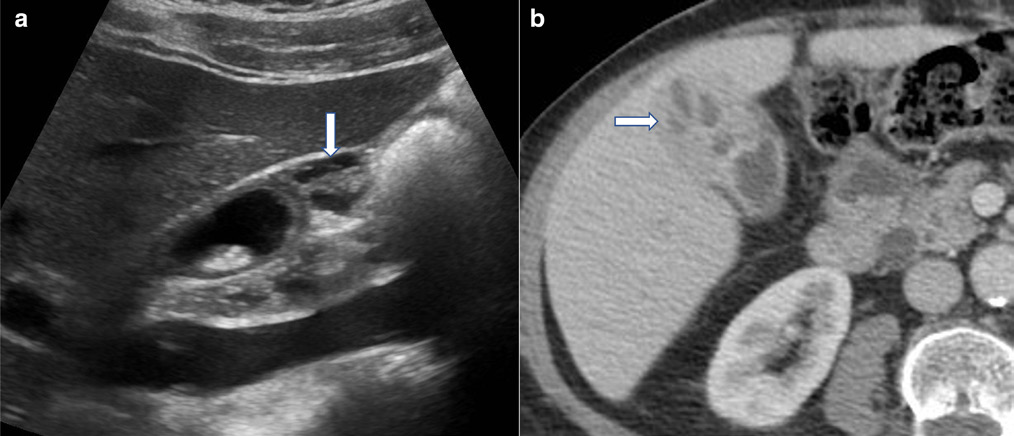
(a) Greyscale ultrasound showing anechoic cystic spaces in a thickened gallbladder fundus consistent with adenomyomatosis. (b) Axial contrast-enhanced CT also demonstrating cystic spaces within a thickened gallbladder fundus. Note the gallbladder mucosa enhances normally and appears intact. The cystic spaces are usually a sign of a benign process and are typical of adenomyomatosis.
